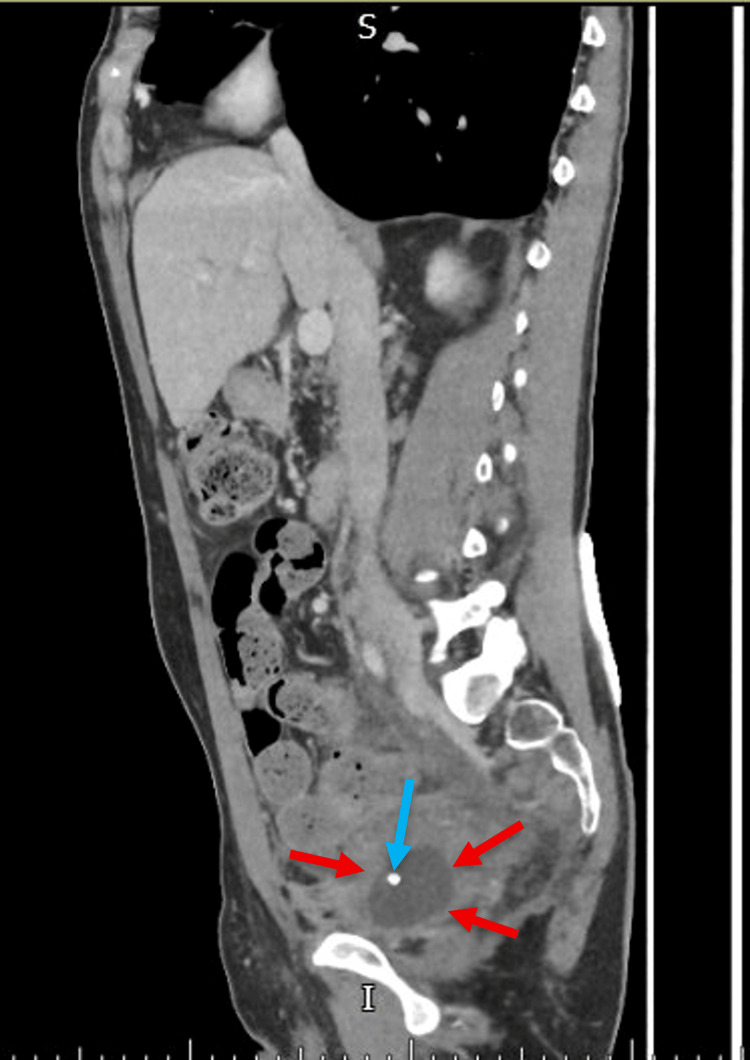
Sagittal contrast CT TAP at initial presentation The urinary bladder demonstrates diffuse wall thickening with a layering enhancement pattern (red arrows). A radiodense focus is identified in the bladder wall, indeterminate for a calculus versus calcification within an underlying lesion (blue arrow). Pericystic fat stranding is present. CT TAP: computed tomography of the thorax, abdomen, and pelvis
